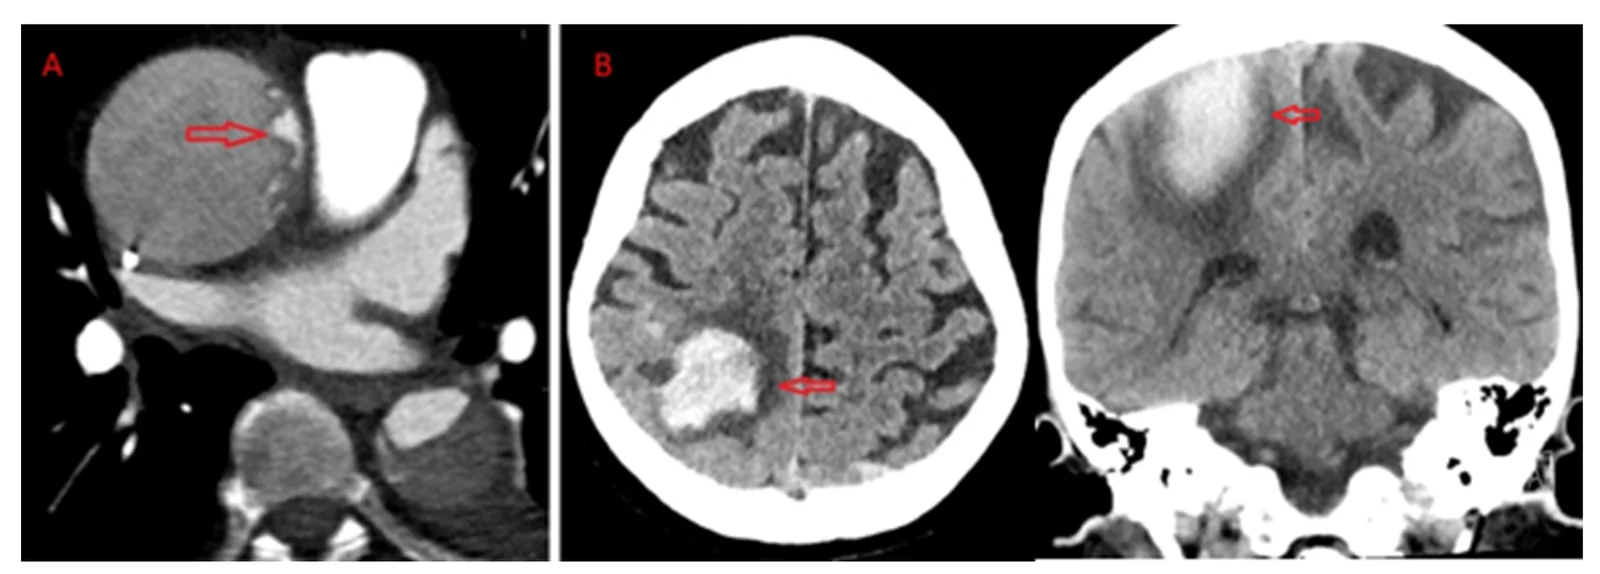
CT imaging two weeks after admission showing stable Stanford type A dissection (A) with enlargement of both cerebral haemorrhages (B).
